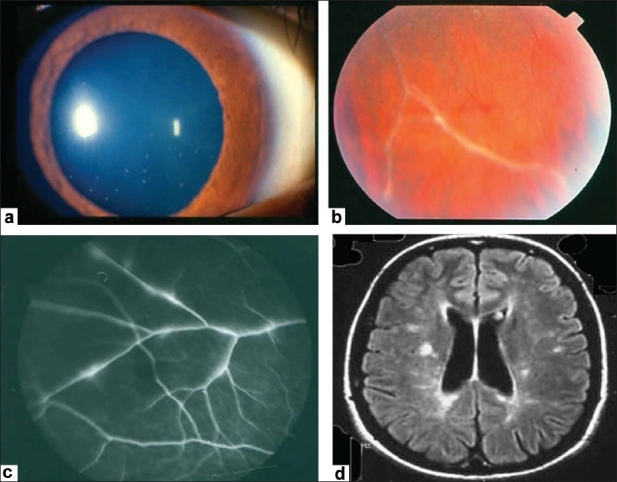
(a) Anterior segment of a 24-year-old woman with multiple sclerosis showing granulomatous keratic precipitates (b) Fundus photograph showing perivenous sheathing (c) Fluorescein angiogram showing focal venous leakage (d) Magnetic resonance imaging of the brain showing demyelinating lesions
